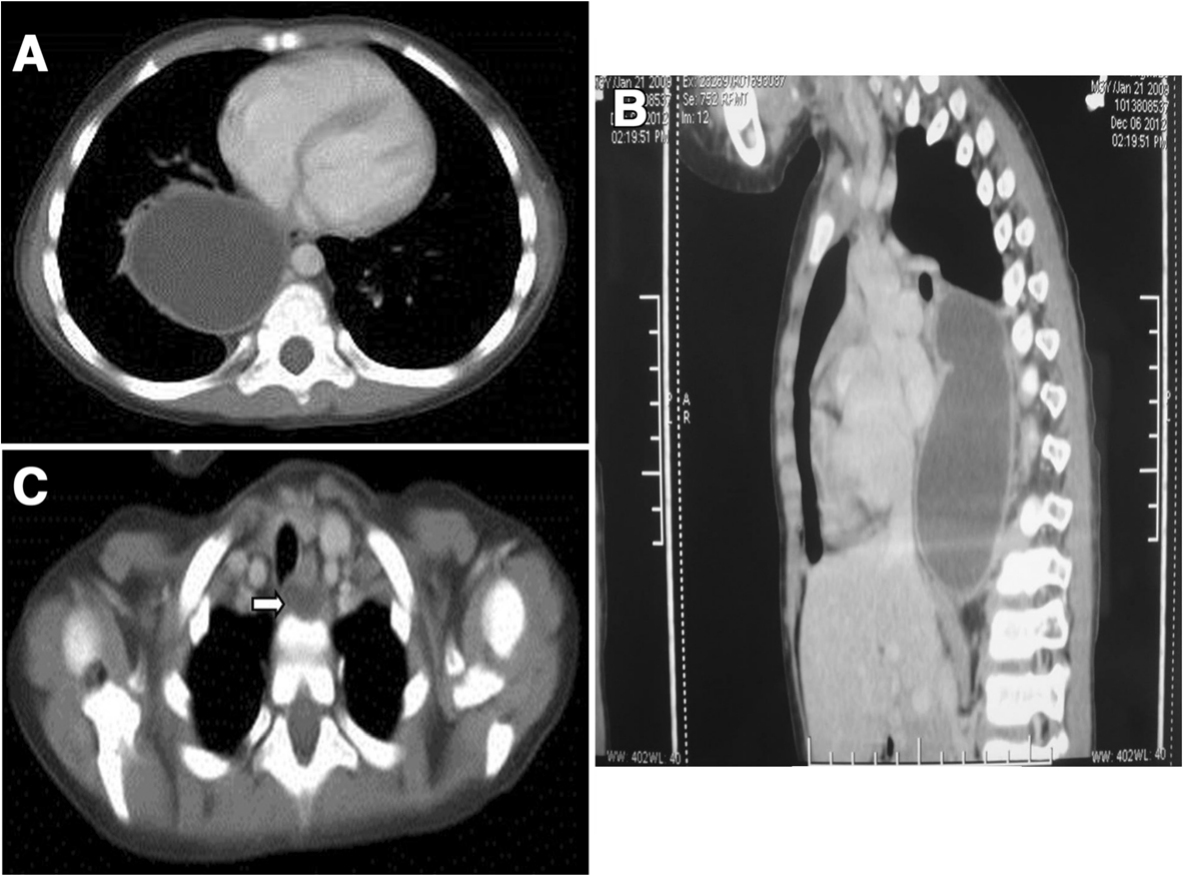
Computed tomography scan of esophageal duplication cysts. (A) Transverse and (B) sagittal views of the large-sized cyst; and (C) transverse view of the small-sized cyst (white arrow).
